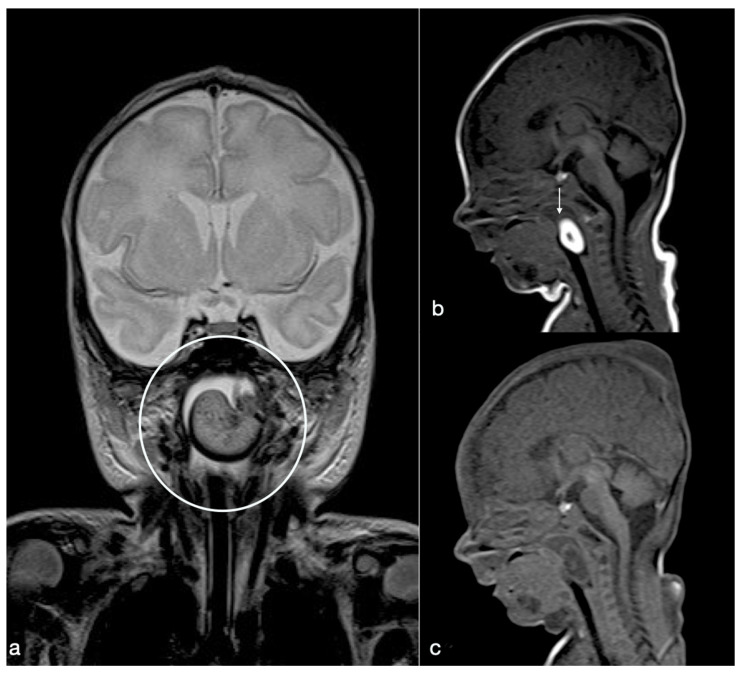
We report the case of respiratory failure in a male newborn, whose pregnancy was unknown until the third trimester when it was discovered due to polyhydramnios. At 38th gestational week, caesarean section was needed due to fetal suffering: he was born pale, floppy, hypotonic, not reagent to external stimuli, with a low heart rate (HR (heart rate) < 60 bpm), not responsive to medical treatment, and showing increasing oxygen desaturation up to respiratory distress. The intubation was challenging, so physicians performed a fiber-optically controlled one, demonstrating a well-defined obstructive nasal-oropharyngeal soft white mass. After vital parameters stabilization, he was immediately transferred to the neonatal intensive care. On physical examination, no abnormalities were noticed; also, cardiac, pulmonary, and abdominal examinations were normal. He promptly underwent magnetic resonance imaging (MRI) investigation to define the lesion. MRI of head and neck revealed a well-defined soft tissue pedunculated mass (2.5 × 2 × 1 cm), arising from the left nasal-oropharynx wall, occupying the space between the epiglottis and the uvula in the midline. In the T2-weighted coronal section (a), it appears hyperintense with small punctate hypointense foci in the center and hypointense peripherally (open circle). A T1-weighted image obtained on the sagittal plane (b) shows a hyperintense “pearly” appearance of the lesion (arrow), with the exception of a small hypointense center. In the unenhanced, fat-saturated sagittal T1-weighted image (c), the mass is completely hypointense, consistent with a fat-rich content. These findings were consistent with oropharyngeal dermoid polyp (DP); in particular, a DP does not show restricted diffusion, contrary to an epidermoid cyst [1]. DPs have to be differentiated by other lesions within the region of the skull base or pharynx, which may cause children’s respiratory distress, such as meningoencephalocele, neuroglial heterotopias, and gliomas [1,2,3]. In particular, a DP differs from a meningoencephalocele, which is hypointense on T1-weighted and FLAIR images (similar to brain tissue), showing a direct communication through a skull base defect with the cranial vault; or from neuroglial heterotopy, which shows T1 and T2 signal characteristics of brain gray and white matter with small enclosed cysts; instead of gliomas, which usually show a hypointense signal on T1-weighted images [1]. Even if the use of ultrasound is useful for the first-line investigation of neck masses in neonates [4], considering the severity of the respiratory failure, our patient directly underwent MRI examination for optimal lesion characterization and surgical planning. Moreover, MRI allowed us to assess the relationship of the lesion with the adjacent soft tissues, to depict adjacent bony changes [3,5], and to exclude intracranial extension, thus providing essential information for the surgical planning [2]. This approach is essential in terms of surgical management and outcome. In our case, there were neither underlying bony skull base defects, nor intracranial extension. No cervical enlarged lymph nodes were found.
